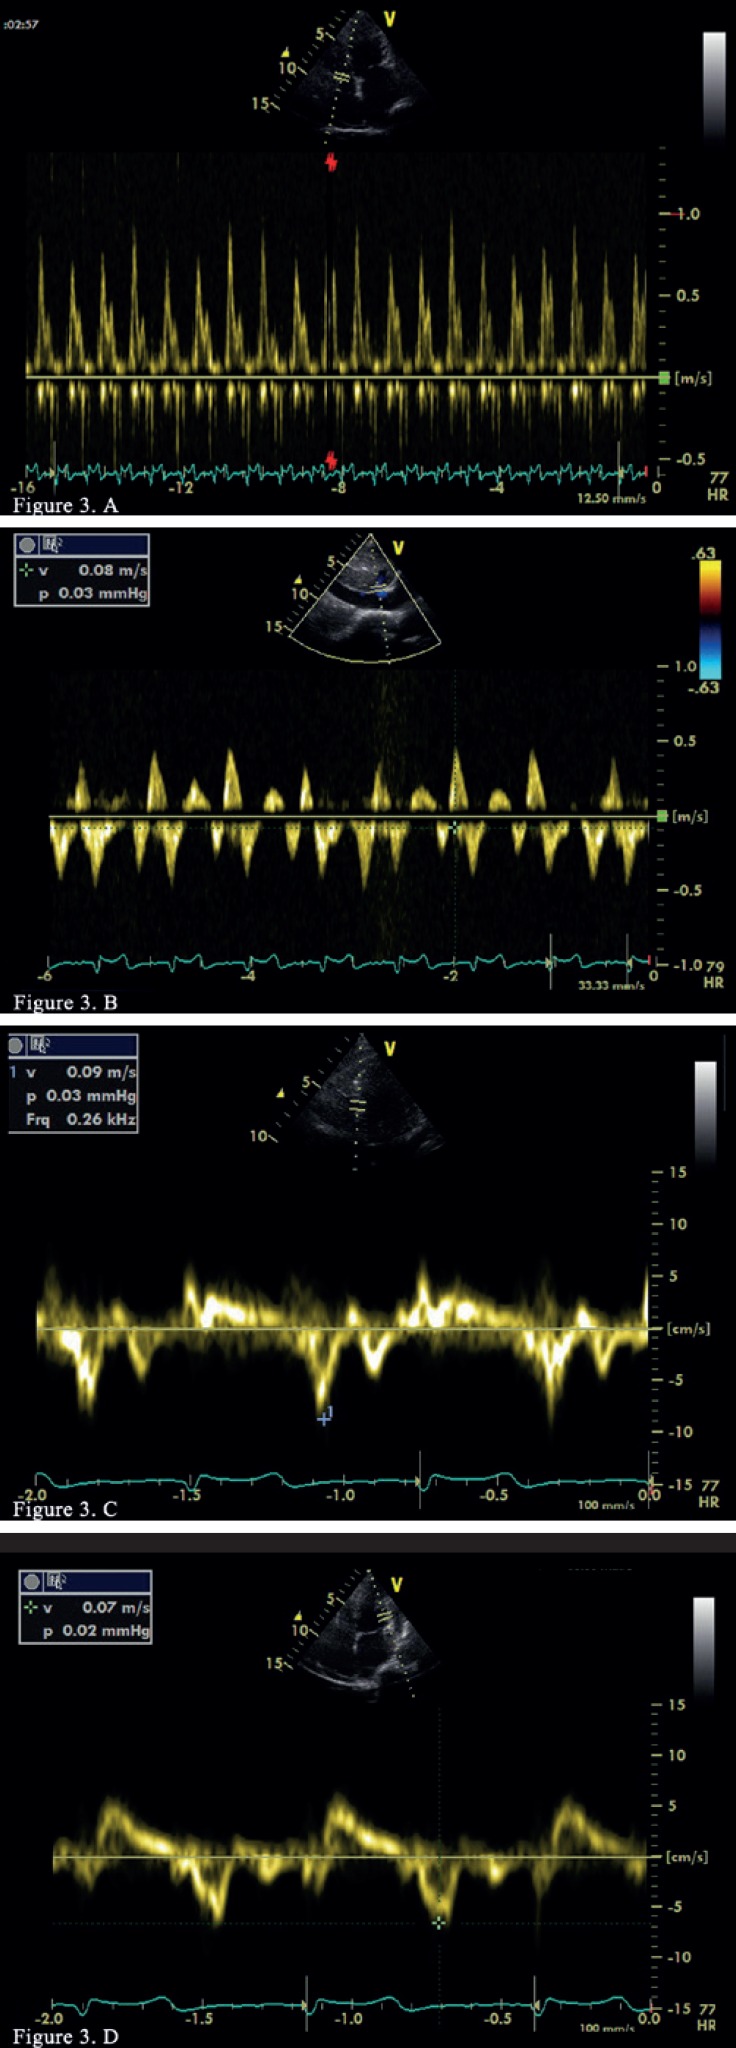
Pulsed-wave Doppler echocardiography demonstrates a restrictive filling pattern and significant respiratory variation in the tricuspid valve inflow (A) and inspiratory diastolic flow reversal in the hepatic vein (B). Tissue Doppler echocardiography reveals annulus reversus (C and D; septal and lateral tissue E' velocity, respectively).
